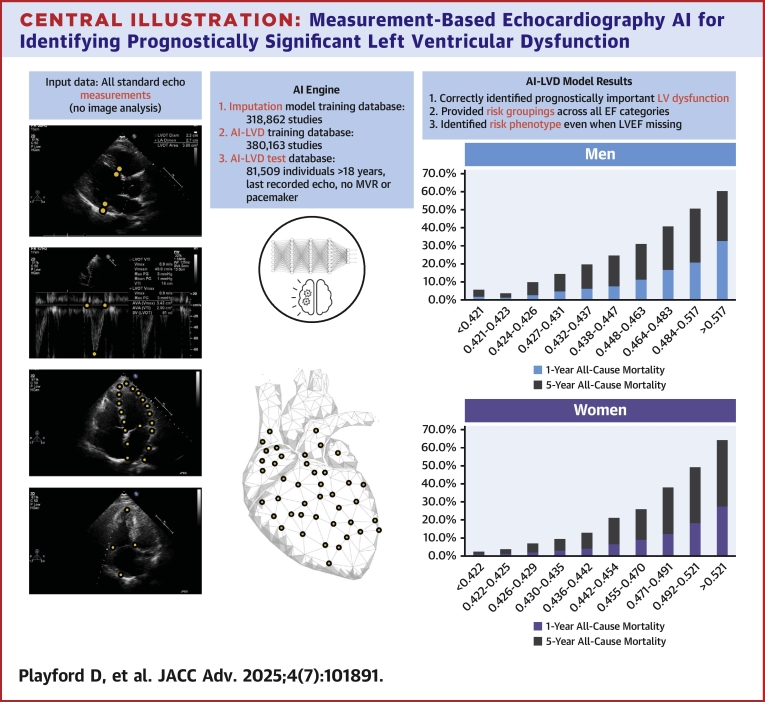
Measurement-Based Echocardiography AI for Identifying Prognostically Significant Left Ventricular Dysfunction Echocardiographic measurement data (no image analysis) indicated in the left panel is taken into the AI model (middle panel), producing a probability output. The right panel demonstrates the unadjusted pattern of actual 1- and 5-year mortality for men (top graph) and women (bottom graph) according to the sex-specific, decile distribution of AI output. LVEF = left ventricular ejection fraction; MVR = mitral valve replacement; other abbreviation as in Figure 1.
